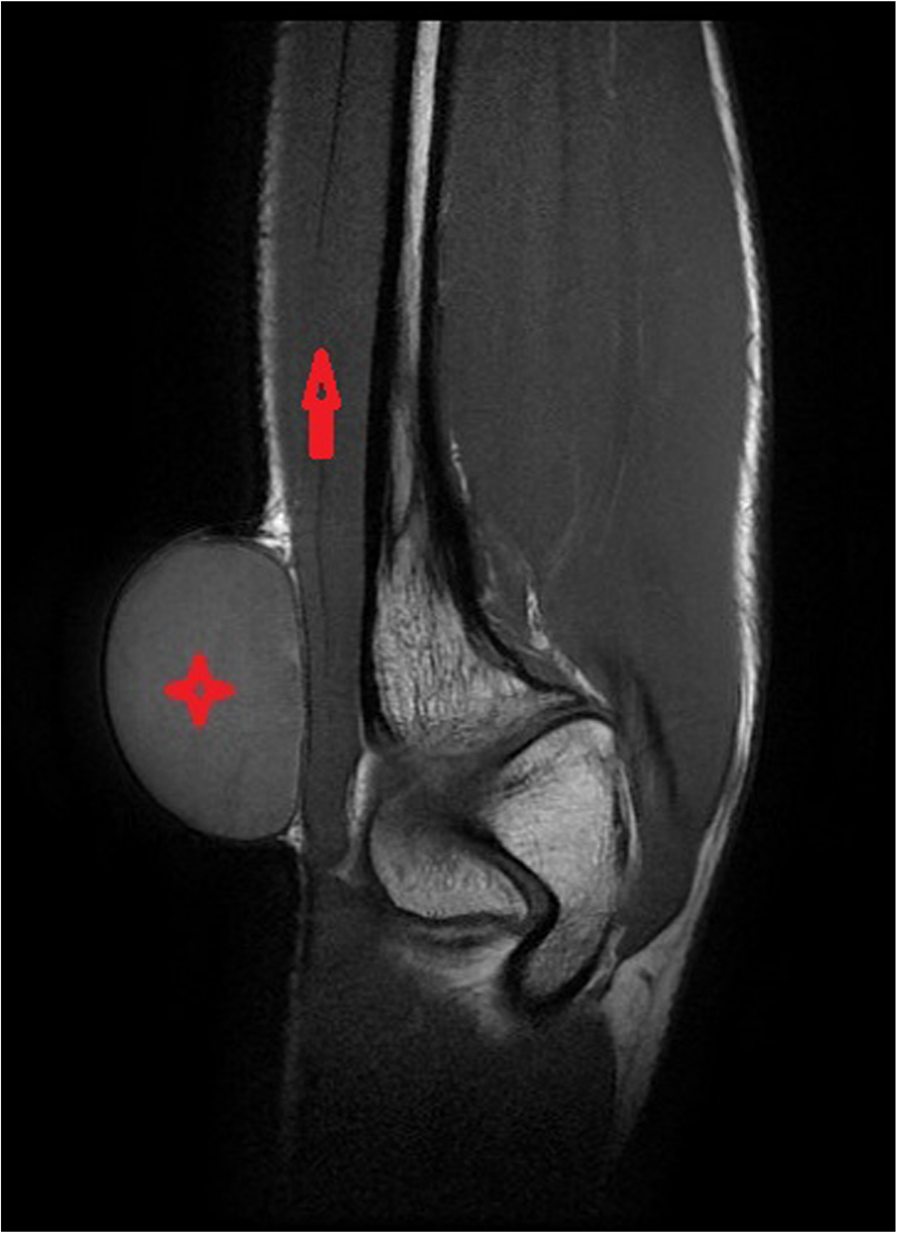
MRI image; T1 coronal view showing cystic lesion with fine capsule () in close relation with flexor carpi ulnaris ()
